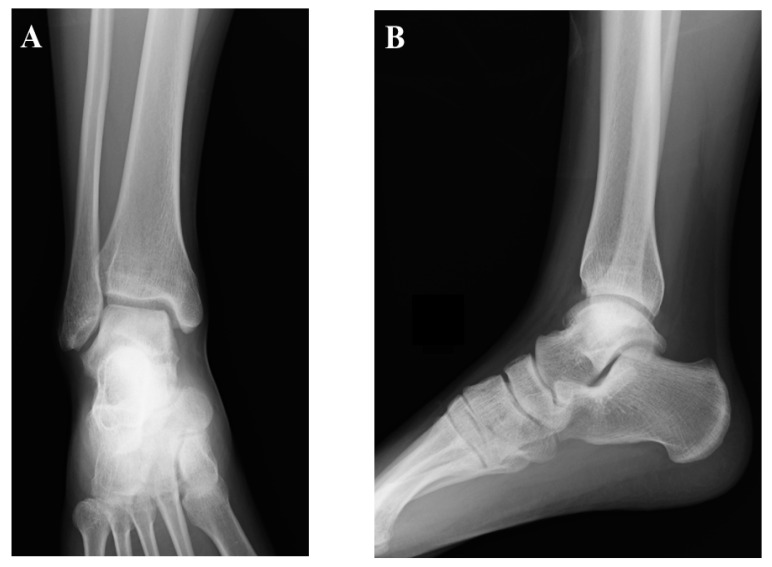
Radiographic findings at 12 months after surgery. (A) Antero-posterior view; (B) lateral view of the right ankle.
